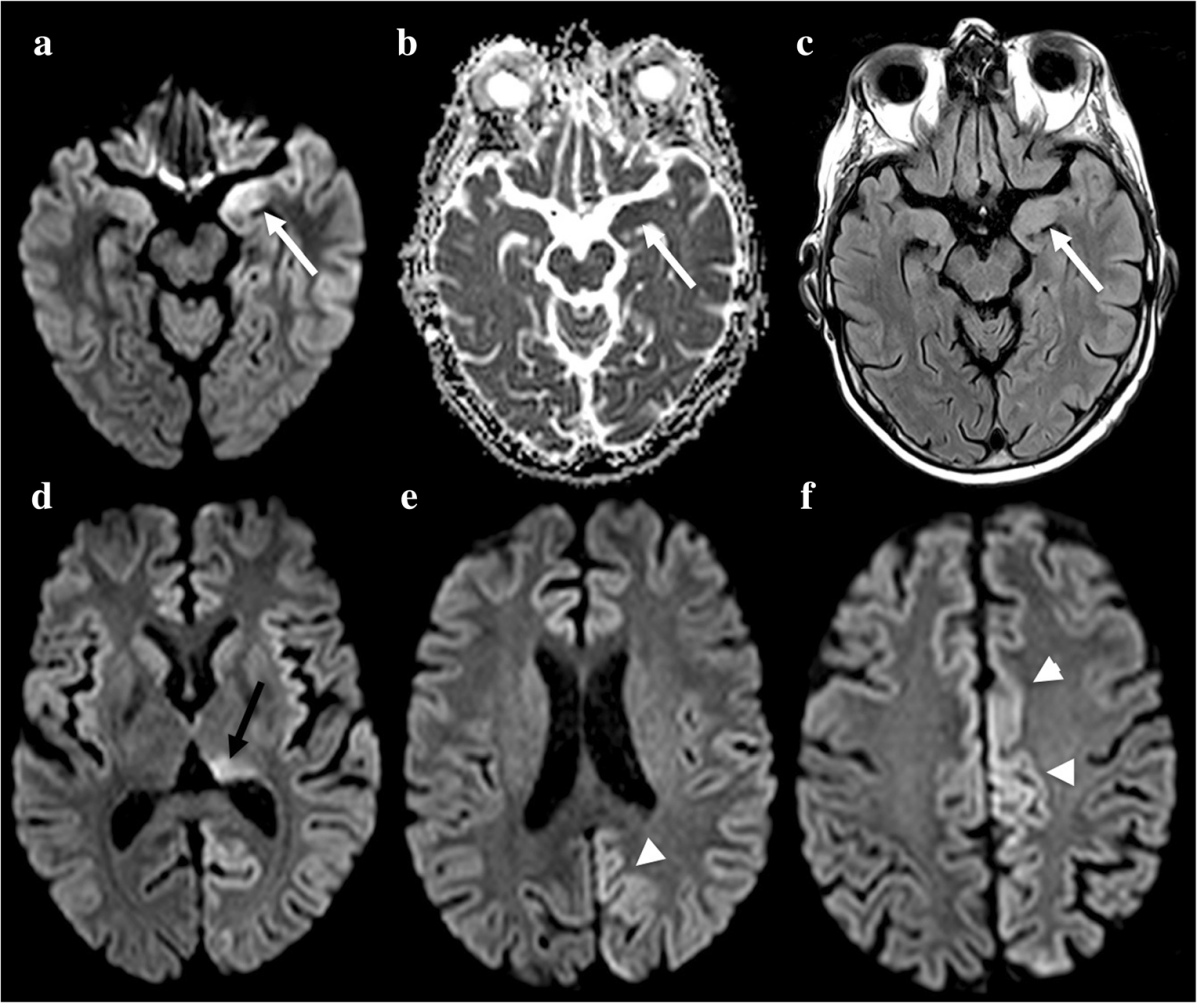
A 50-year-old man with status epilepticus presenting with aphasia and right hemiparesis in a context of chronic alcoholism. Hyperintensities on DWI (a, d, e, f) and FLAIR (c) with subtle restriction on ADC (b) of the left hippocampus (white arrow), pulvinar (black arrow) and cortex (arrowheads)
